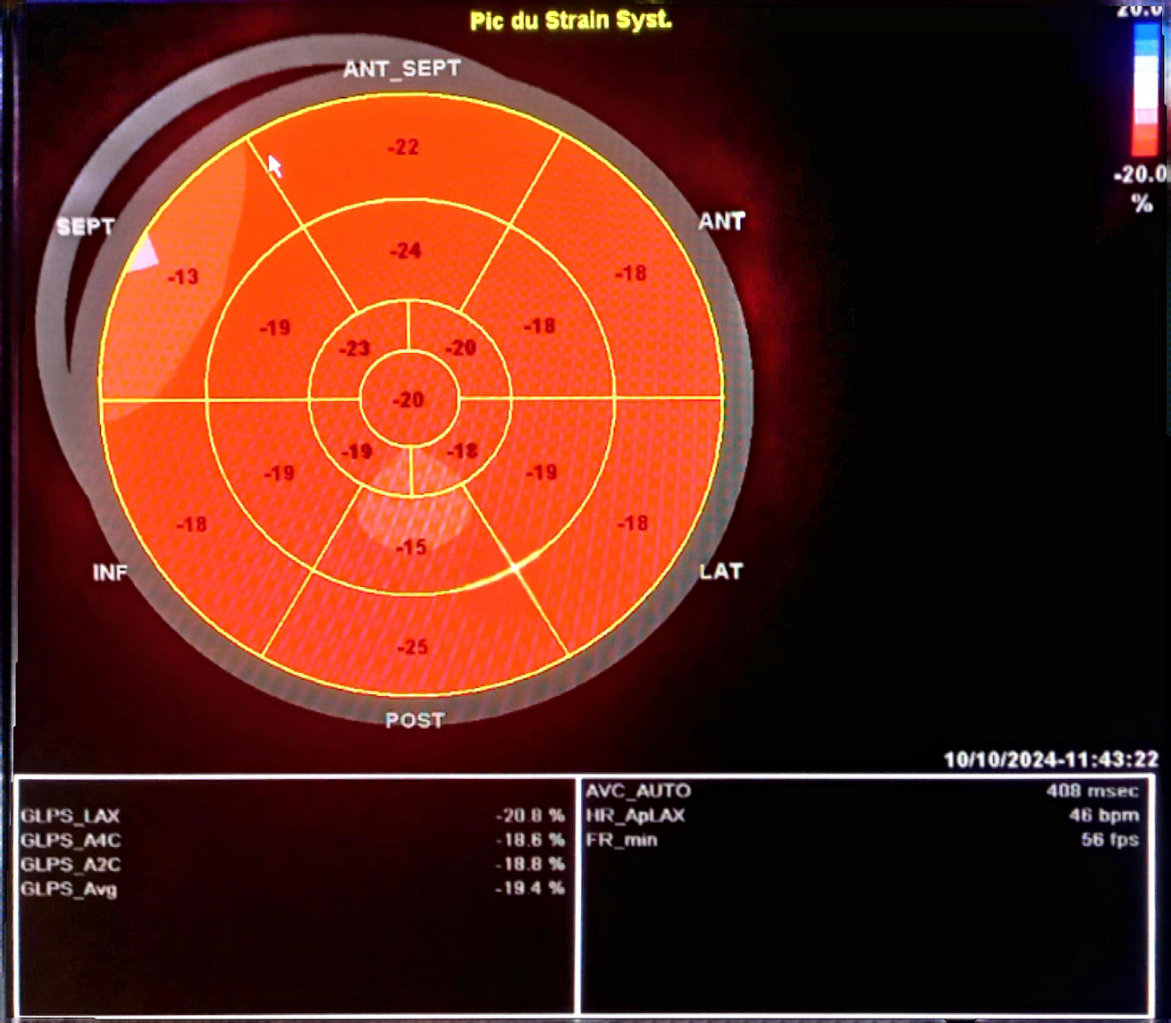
Echocardiography (ETT) showing improvement in global and segmental systolic function with a global longitudinal strain of −19.4%.
